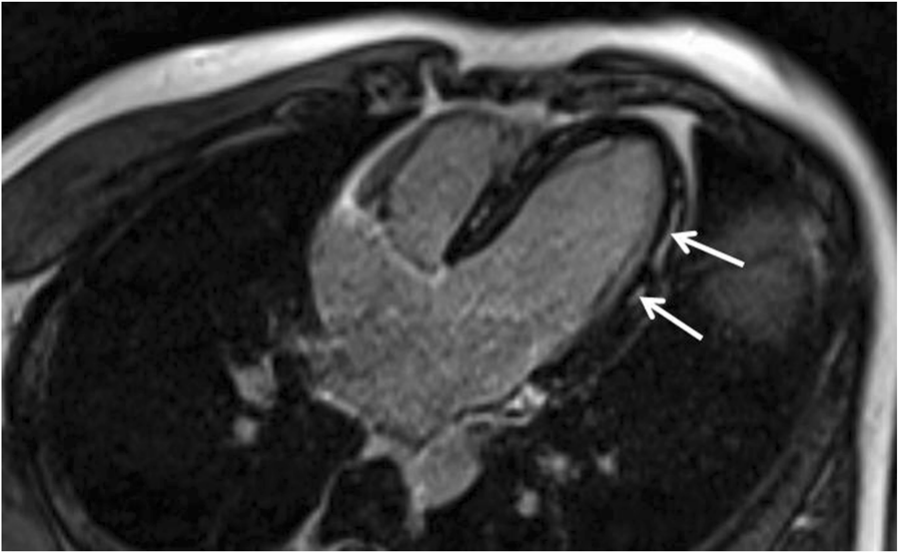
Typical magnetic resonance imaging image of myocarditis. This figure shows a magnetic resonance imaging four chamber cardiac view. Mid-wall late gadolinium enhancement of the lateral wall is visible (arrows). This is a common MRI aspect of myocarditis.
